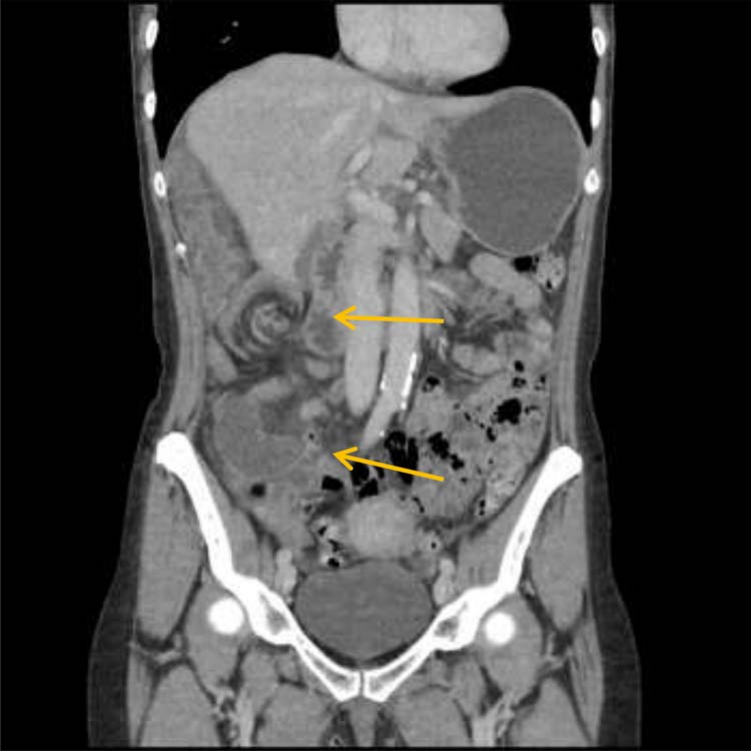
CT scan shows a vascular/mesenteric pedicle swirl suggestive of malrotation or volvulus with evidence of dilated closed loop obstruction.
